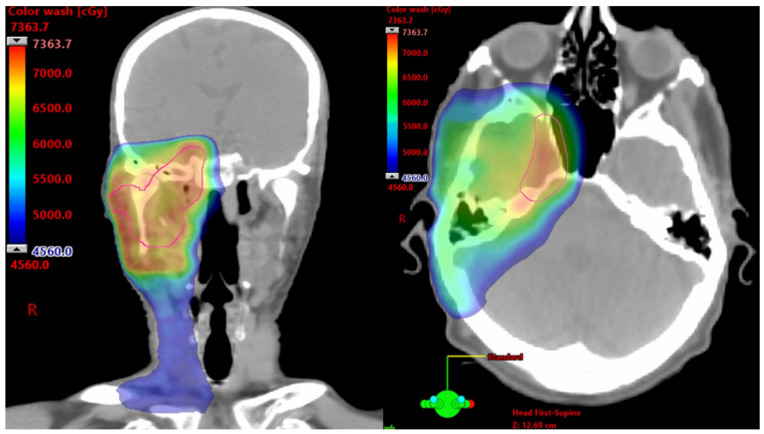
Axial and Coronal Images From a Computed Tomography Planning Scan. Color wash dose distribution of a patient receiving 7320 cGy using a 120 cGy twice/day hyperfractionated approach to the right skull base and elective right neck.
